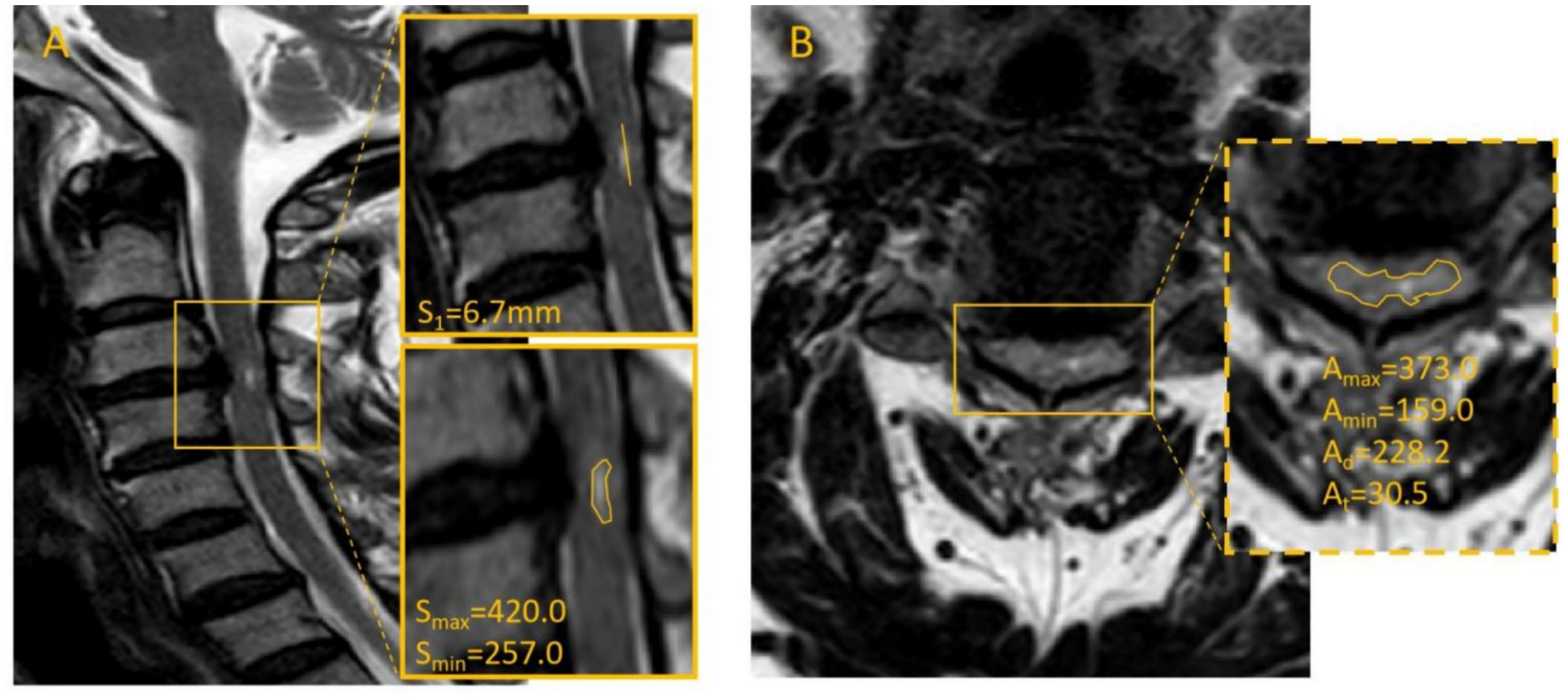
Patient Wu, male, 45 years, admitted 5 days after a motor-vehicle accident. The patient presented with hyperesthesia and decreased muscle strength predominantly in the left limbs; the admission JOA score was 9. The admission cervical MRI is shown in A and B; the measured axial–sagittal difference ratio was 131.29%. The patient underwent single-level ACDF. At 3 months postoperatively, the JOA score was 12 and the RR was 37.5%; at 2 years postoperatively, the JOA score was 16 and the RR was 87.5%.
